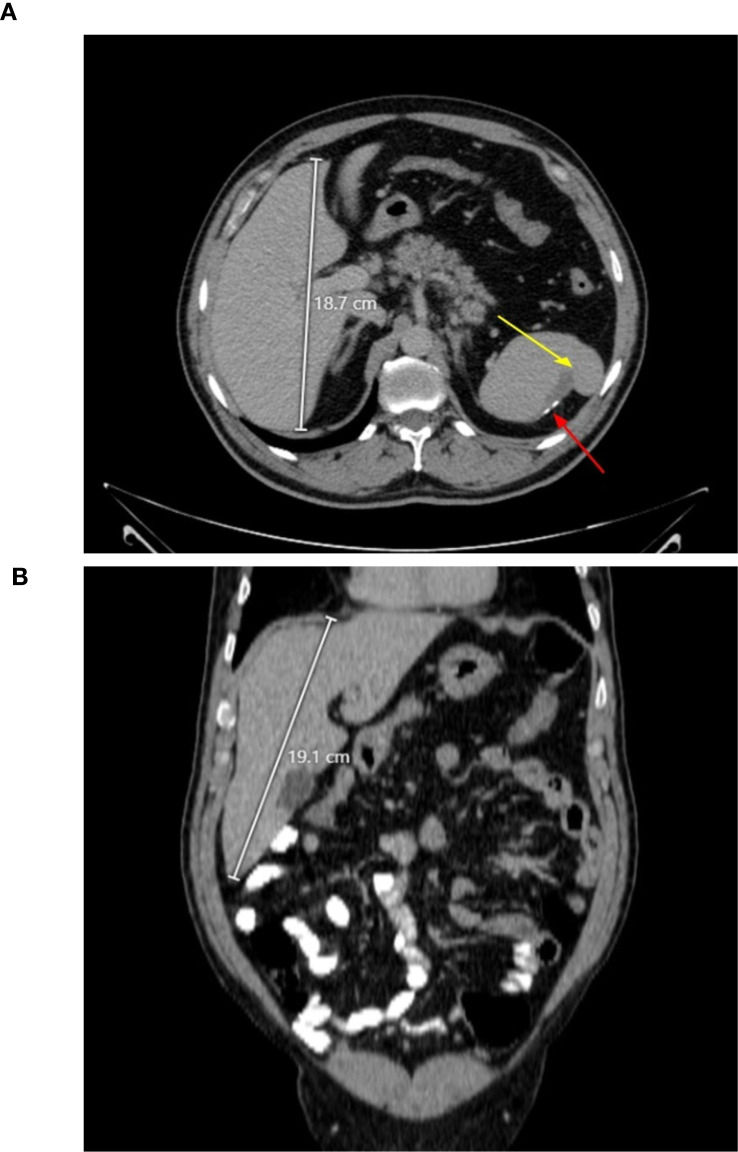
Axial (A) and coronal (B) abdominal CT scan demonstrating hepatomegaly. The spleen appears normal in size. (A) A hypodense splenic lesion (yellow arrow) indicates prior infarction; foci of calcification (red arrow) are consistent with post-infarct changes. (B) The liver measures 19.1 cm in maximal craniocaudal dimension (white line), consistent with significant hepatomegaly.
